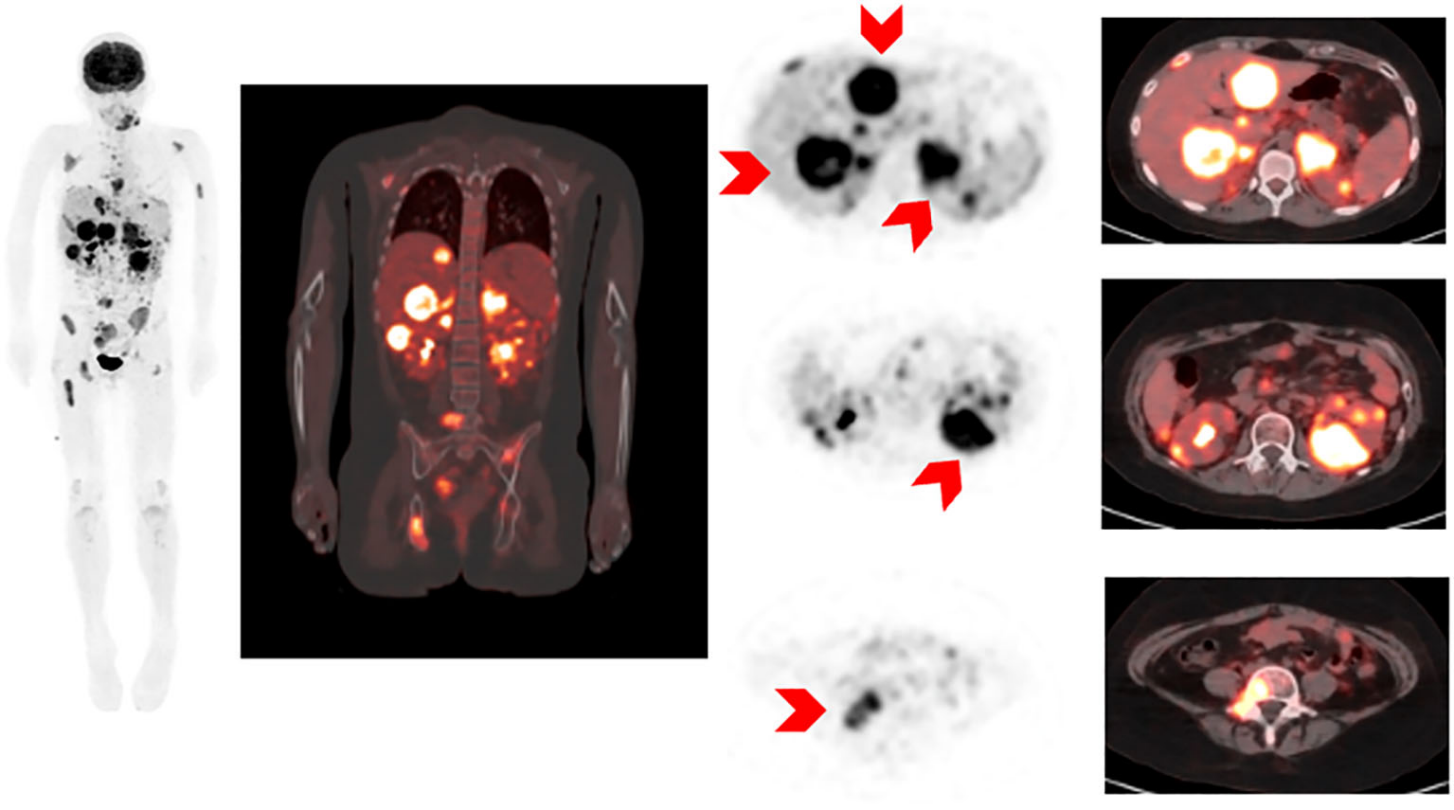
18F-FDG PET/MRI MIP (maximum intensity projection image) and selected axial images of 18-FDG PET and fusion PET images and MRI images showing diffuse abnormal 18F-FDG activity involving liver, kidneys, retroperitonal nodes, bowel and peritoneal implants, bony pelvis.
